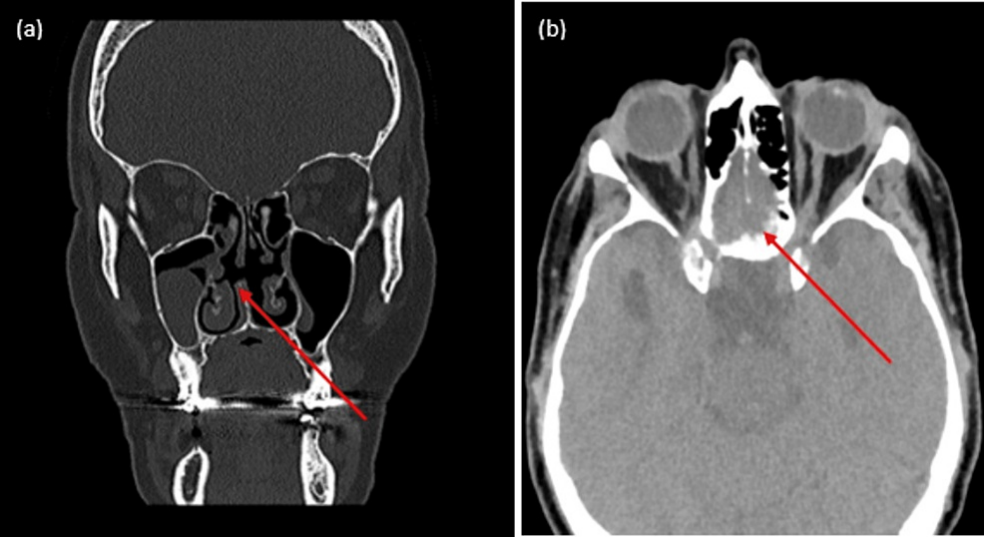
CT sinuses with coronal (a) and (b) axial cuts showing bilateral opacification (red arrows)
